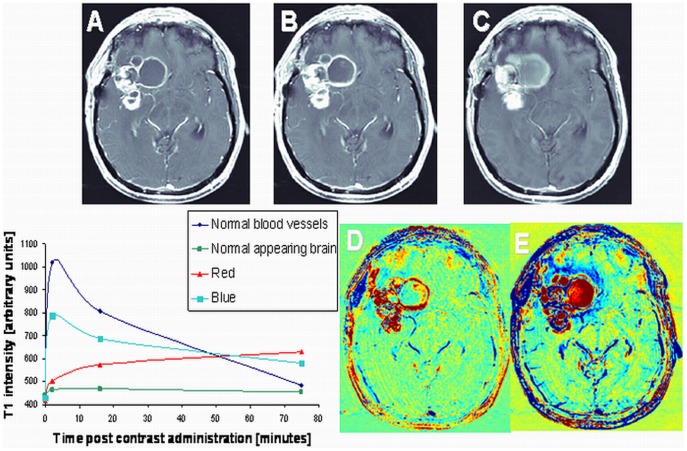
Enhancement subtraction maps. Examples of axial high resolution T1-weighted MR images acquired 2 min (A), 15 min (B) and 75 min (C) after contrast administration in a patient (#3) with newly diagnosed GBM undergoing standard chemoradiation are shown. Subtraction maps were calculated from the data acquired at 2 and 15 min (D) and 2 and 75 min (E) post contrast administration. Blue regions represent fast clearance of the contrast agent from the tumor while red regions represent slow accumulation of the contrast in the tissue. It can be seen that abnormal enhancement patterns in the 75 min map are depicted more clearly and over larger regions than in the 15 min map. The signal intensity of regions with different enhancement patterns as a function of time post contrast administration is shown in the plot. It can be seen that the red and blue components of the tumor enhance and decay at different rates.
